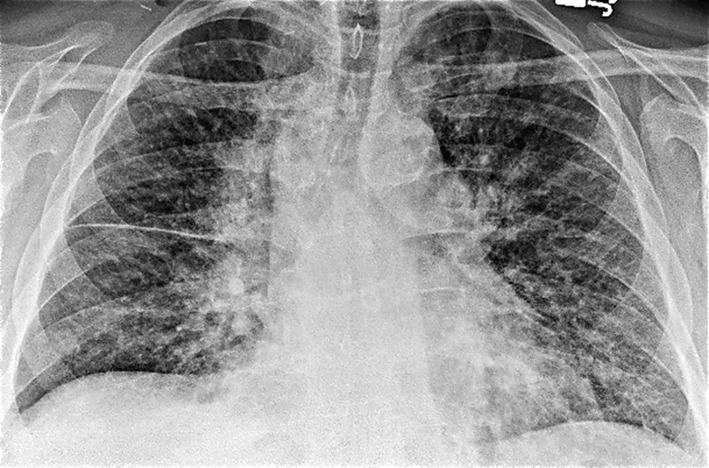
Chest X-ray on admission showed bilateral perihilar haziness and fluid in right transverse fissure consistent with pulmonary edema.
